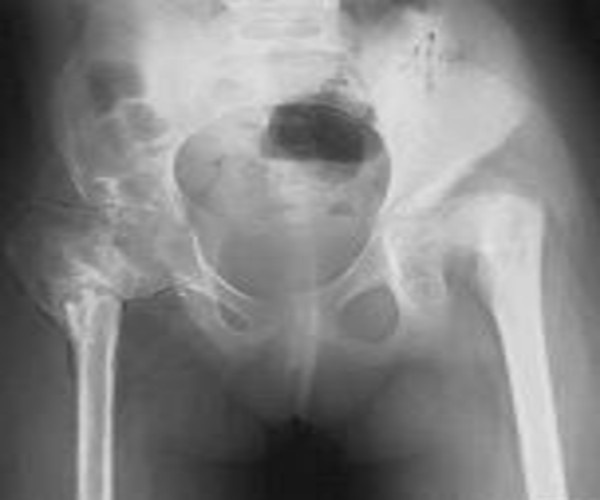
(Patient II). Anteroposterior pelvis radiograph showed massive osteolysis crosses the epiphyseal growth centers of the proximal femora bilaterally, resulting in growth disturbance with radiological appearance resembles the sucked end of a candy-sugar stick, severe osteolysis of the proximal femora were present, the right hip was completely ankylosed with severe generalized osteopenia, progressive osteolysis associated with rudimentary cortices.
